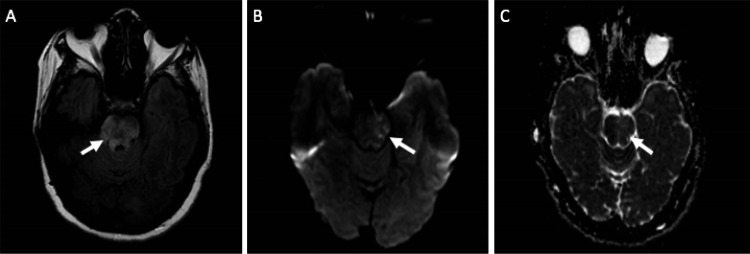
MRI scans from the initial presentation. Arrows in each panel indicate the pontine region of interest. (A) Axial T2 fluid attenuated inversion recovery imaging (B) Axial diffusion weight imaging (C) Axial apparent diffusion coefficient imaging
